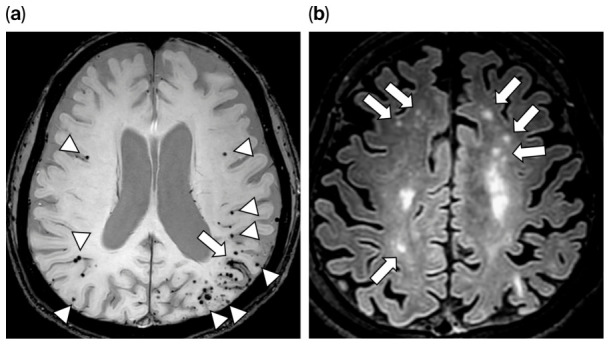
A representative CAA case in a 74-year-old man (MMSE score, 27). (a) SWI-P shows multiple CMBs (arrowheads) in both hemispheres, and cSS (arrow) in the sulcus of the left parietal lobe. (b) 3D-FLAIR shows WMH multi-spot patterns (arrows) in both hemispheres.
